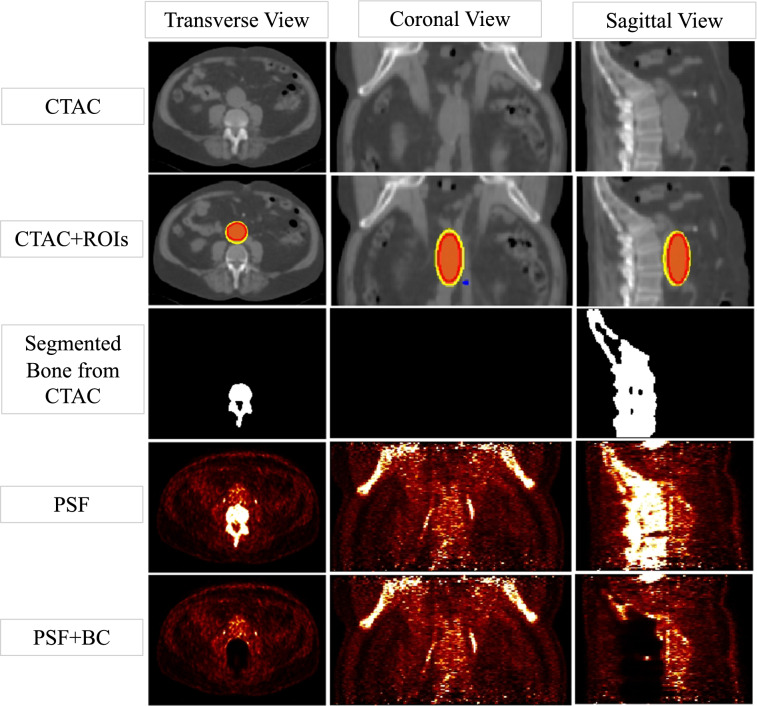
CT images and PET-reconstructed images of a patient dataset, showing a high [^18^F]-NaF uptake in the bone and the aneurysm. The activity contribution from the bone was removed using PSF+BC. The ROIs used to extract the SUVs at the aneurysm are shown on the CTAC image. The outer yellow and inner red ROIs represent AAA and AAA_exc_, respectively. Following past research,^15^ AAA_exc_ was drawn such that its distance from the bone is approximately 4 mm. The blue small spherical region highlights the background ROI used for blood pool correction and the calculation of TBR
